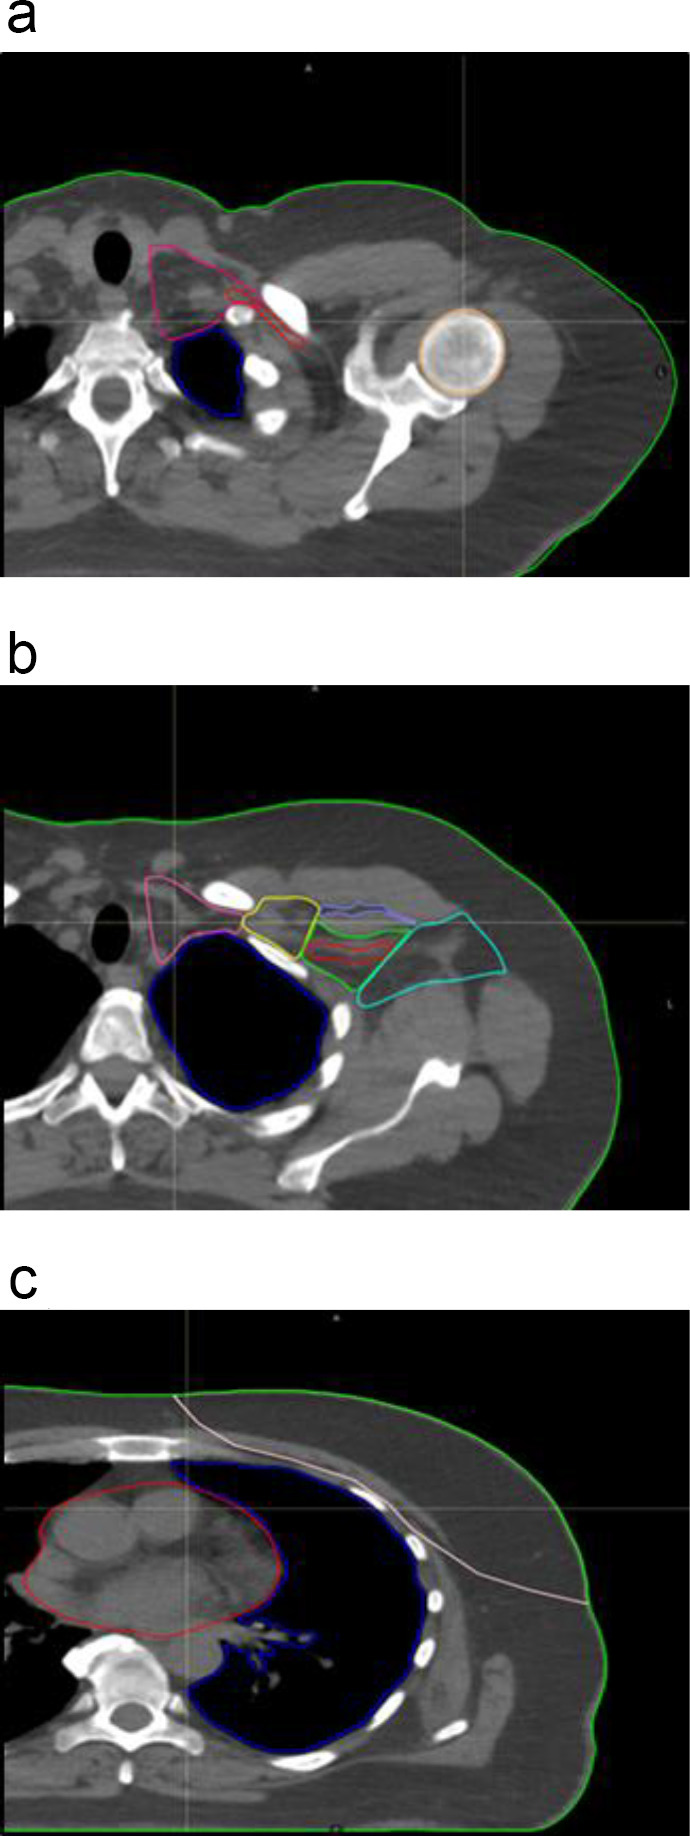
Typical CT axial slice images with manual segmentations of normal and nodal structures utilised during radiotherapy planning. a) demonstrates the level 4 nodes (pink), humeral head (orange), brachial plexus (red), and lung (blue). Figure 1b) level 4 - (pink), level 3 - (yellow), level 2 - (green), level 1 - (aquamarine), and inter-pectoral nodes (lilac), brachial plexus (red), and lung (blue). Figure 1c) lung (blue), heart (red), and breast clinical target volume (salmon).
